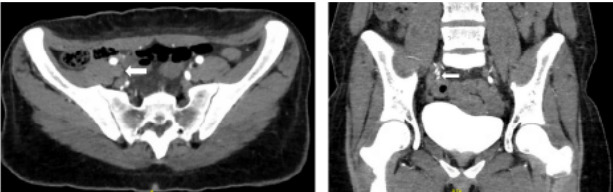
Right internal iliac artery thrombosis indicated by white arrow on computed tomography angiography—axial view on the left and coronal on the right—done in the setting of epigastric pain.
